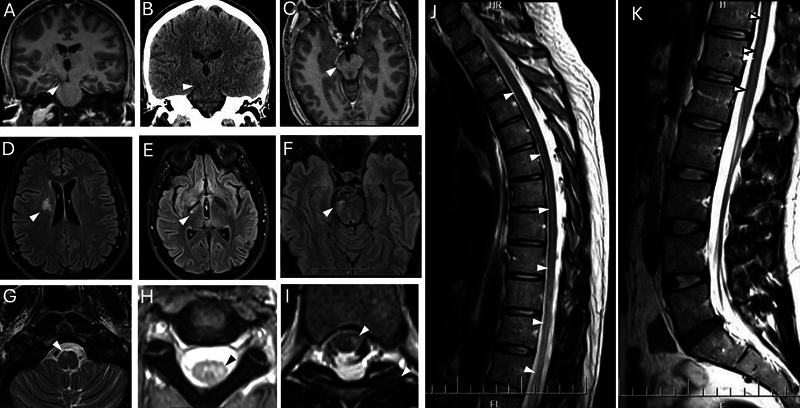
Extensive Wallerian degeneration of corticospinal tract secondary to intracranial germinoma. Coronal post-contrast T1 magnetic resonance imaging (MRI) brain exhibiting hypointensity along the right corticospinal tract from internal capsule to brainstem (
